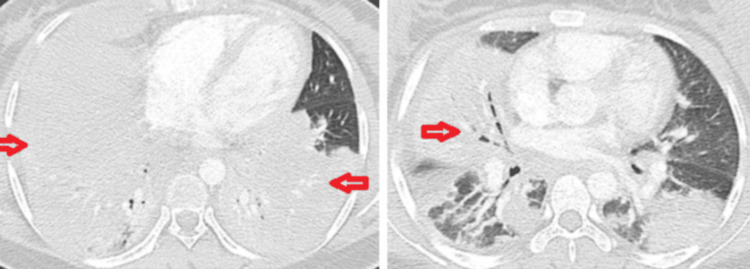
Chest CT at admission. Well-defined areas of consolidation in the bilateral lower lobes, the right middle lobe, and the lingular segment, with air bronchograms and bilateral peribronchovascular thickening. A mosaic perfusion pattern is also noted, with alternating areas of hyper and hypoattenuation.
